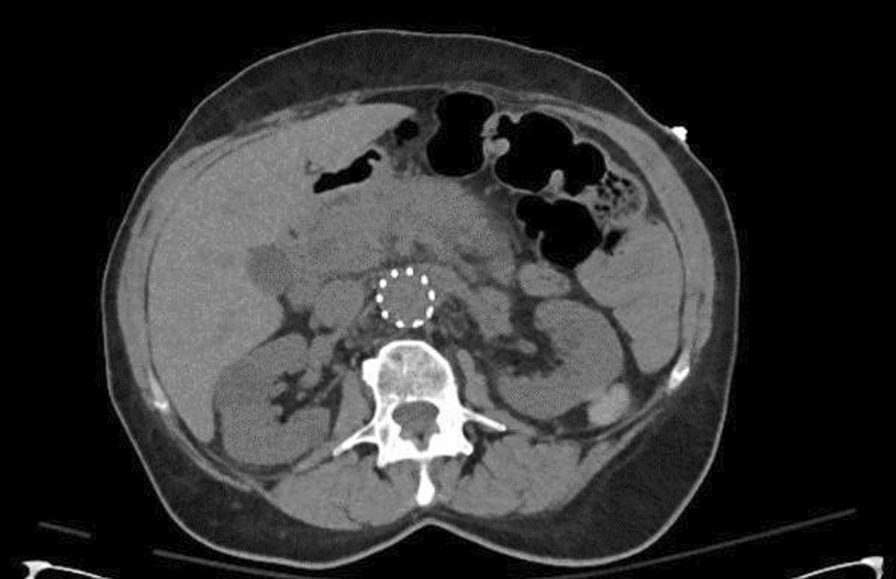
Computed tomography of the abdomen showing acute pancreatitis features
